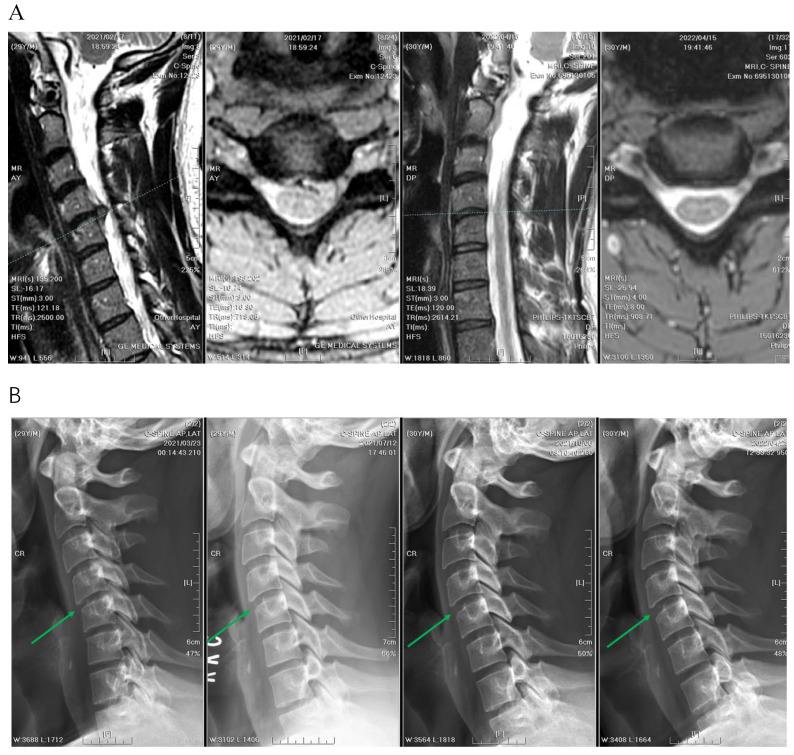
(A) The intervertebral disc height of C4–C5 decreased slightly from 4.4 mm to 4.1 mm, before and after PPECD (MRI before PPECD on the left and 1 year after the surgery on the right). (B) The images from left to right are radiographs before PPECD and 3 months, 6 months, and 1 year after the surgery. Green arrows point to the C4–C5 disc space.
